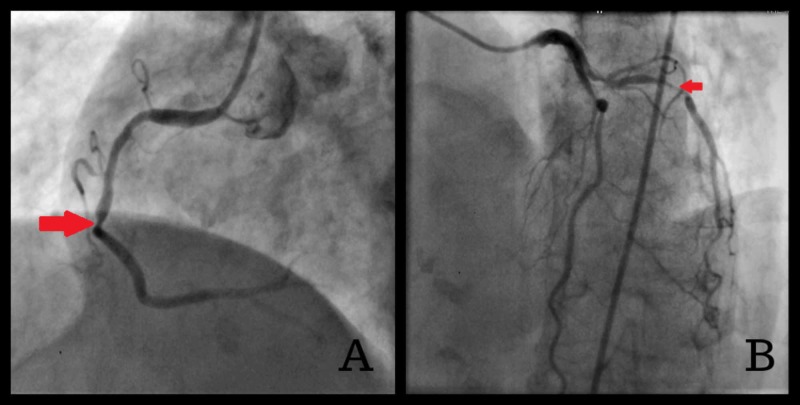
Coronary CT angiography images on admission (A) marked occlusion of mid-RCA (B) in-stent restenosis of the LAD CT, computed tomography; RCA, right coronary artery; LAD, left anterior descending artery.
